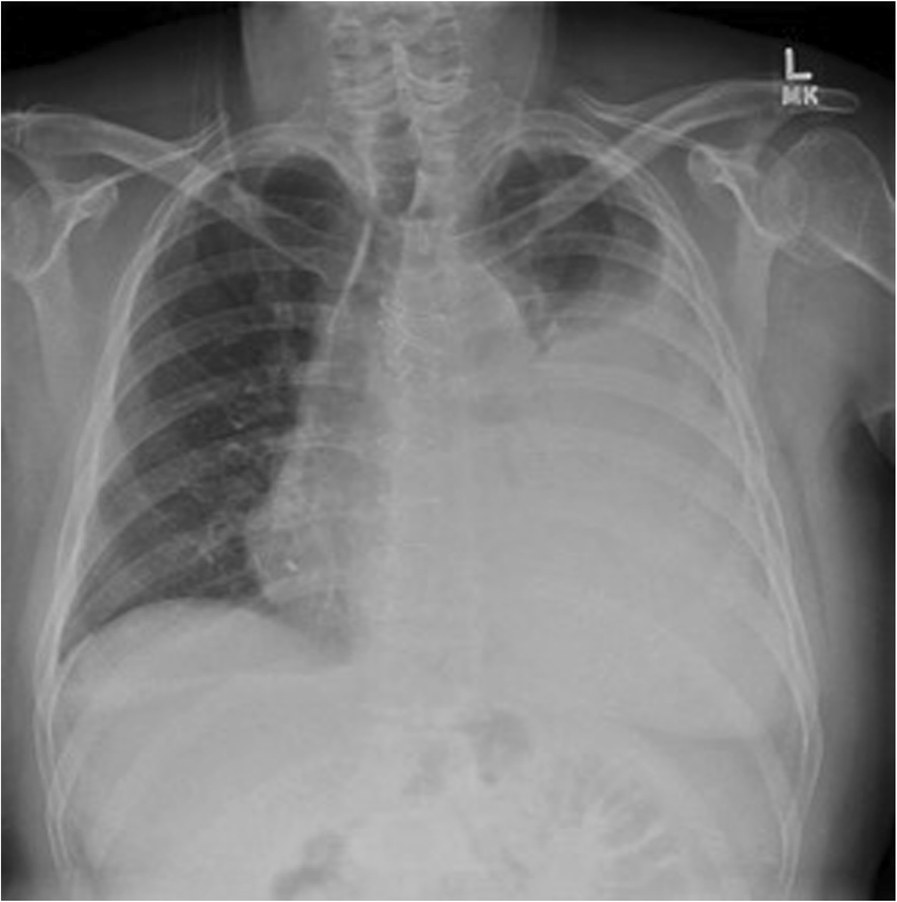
Chest radiograph detected a large pleural effusion over the left lung field on post-operative day 13 after coronary artery bypass grafting
